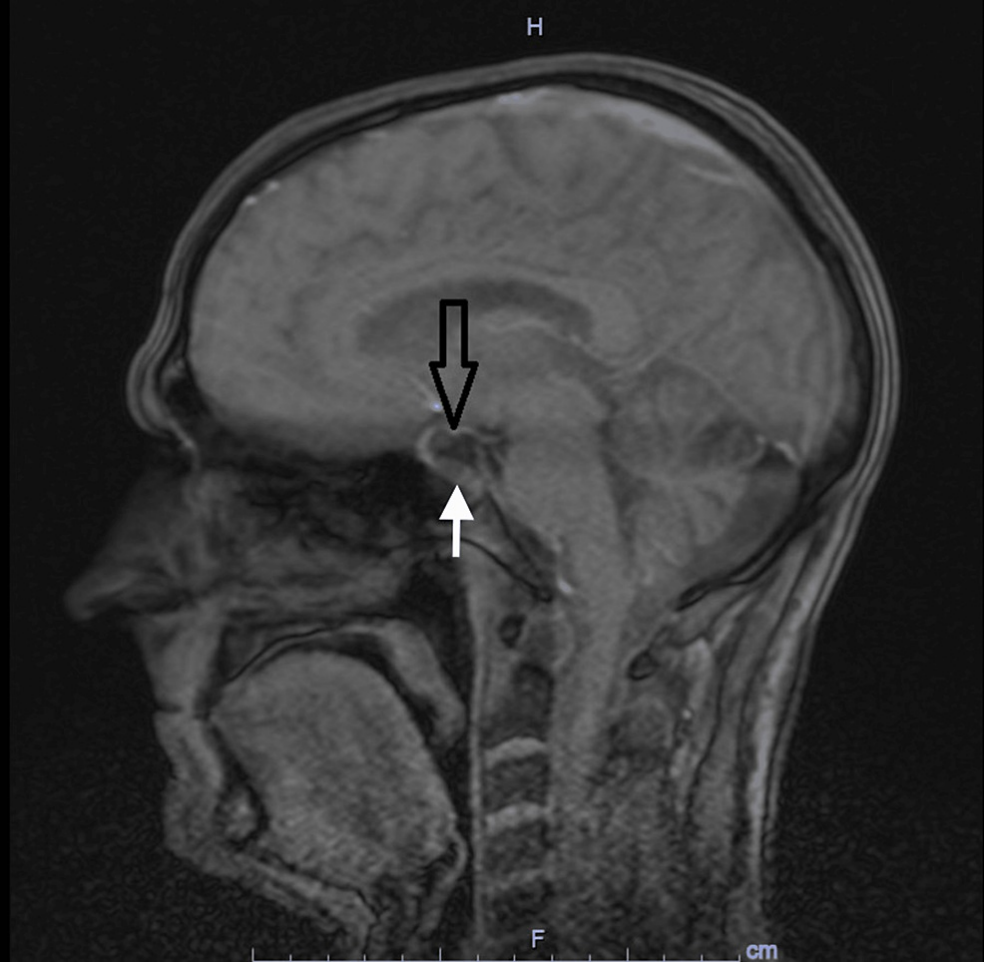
Magnetic Resonance Imaging (MRI) Brain MRI brain showing partially empty sella turcica syndrome ( black arrow) with a small nodule at the floor of the turcica (white arrow).
